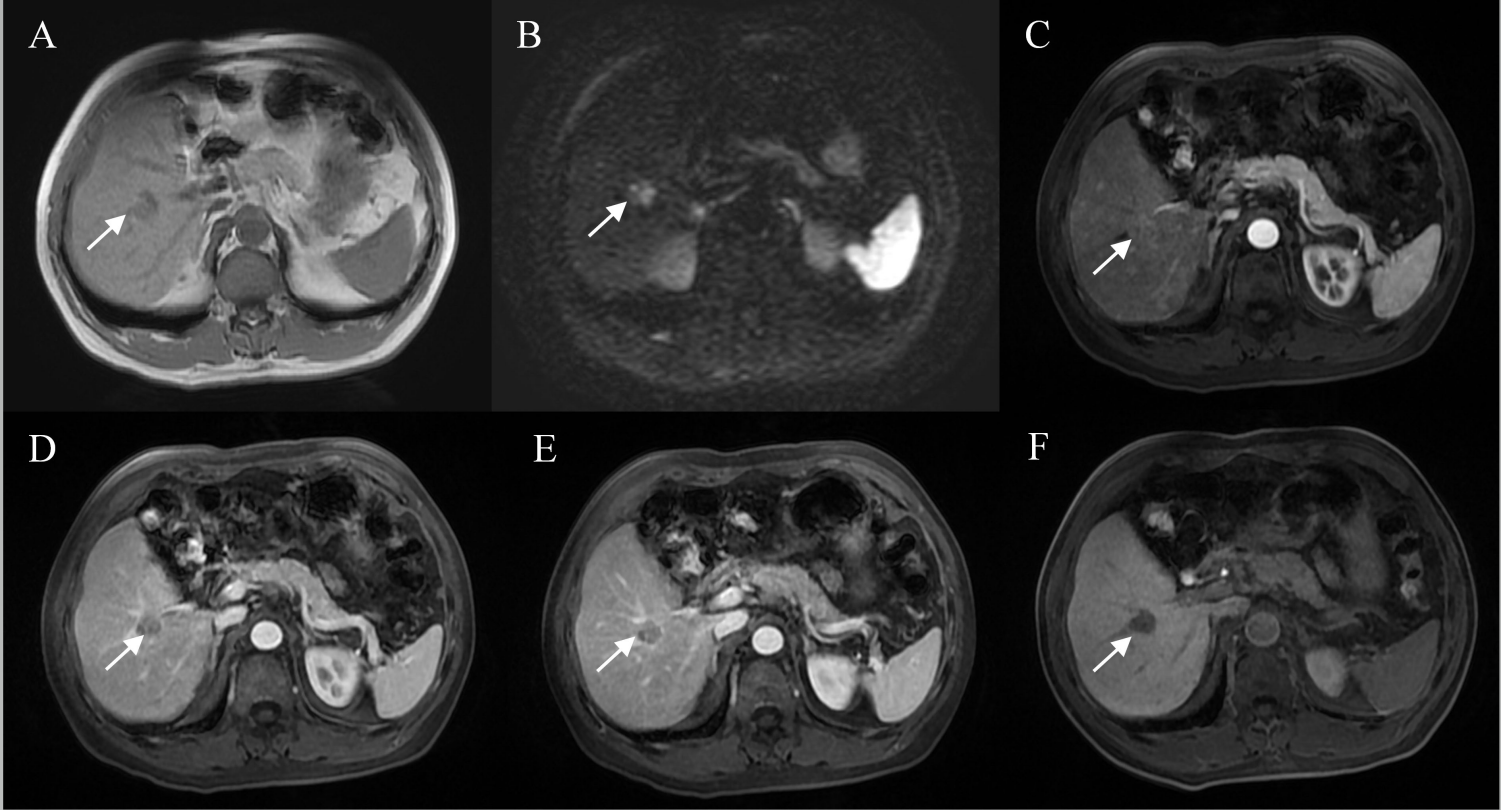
Representative images of MVI positive cases: A 60-year-old male with elevated AFP, Gd-BOPTA MRI detected a solid lesion in hepatic segment V (A), restricted diffusion (B), atypical enhancement pattern without “wash-in” (C–E), incomplete capsule enhancement on portal venous phase and transitional phase images (D, E), and homogeneous HBP hypointensity (F).
